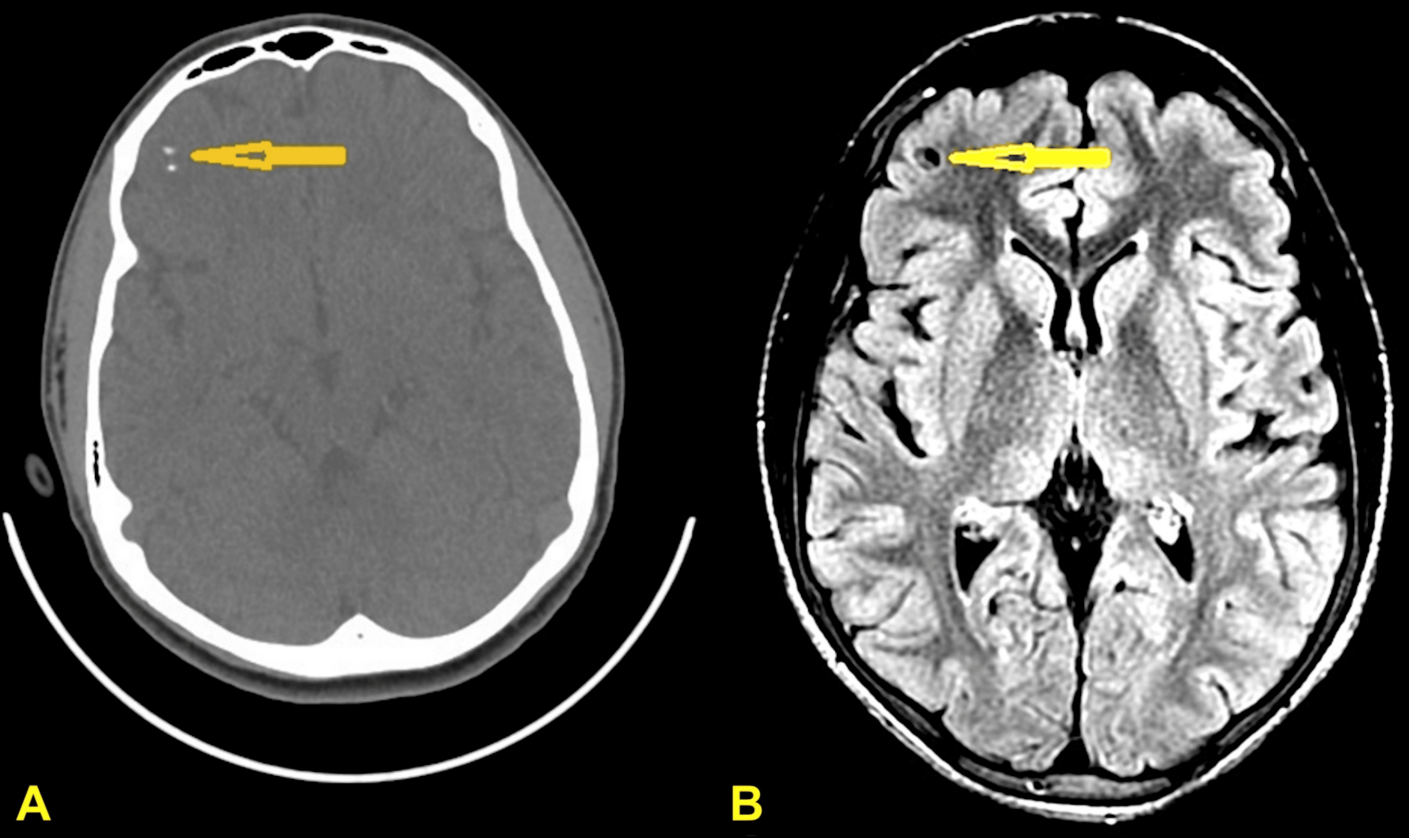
CT and MRI imaging of neurocysticercosis (A) Initial CT scan reveals calcifications within the right frontal lobe, consistent with chronic or inactive neurocysticercosis. (B) Initial MRI (T2-weighted and FLAIR sequences) identifies a 6.1 mm cystic lesion in the right frontal subcortical region, with a visible scolex along the wall—features consistent with a vesicular-stage lesion. These imaging findings fulfill the major neuroimaging criteria for neurocysticercosis, as defined in established diagnostic frameworks. FLAIR: fluid-attenuated inversion recovery; MRI: magnetic resonance imaging; CT: computed tomography
